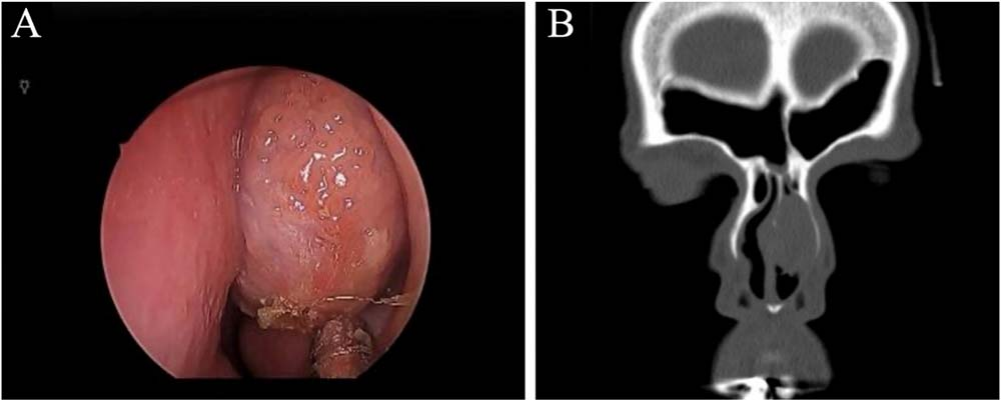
(A) Nasal endoscopic examination showing left nasal septal mass. (B) Computed tomography scan of the paranasal sinuses showing a focal soft tissue lesion arising from the left deviated nasal septum, causing thinning of the left nasal bone measuring 2.2 × 1.7 × 2.7 cm.
